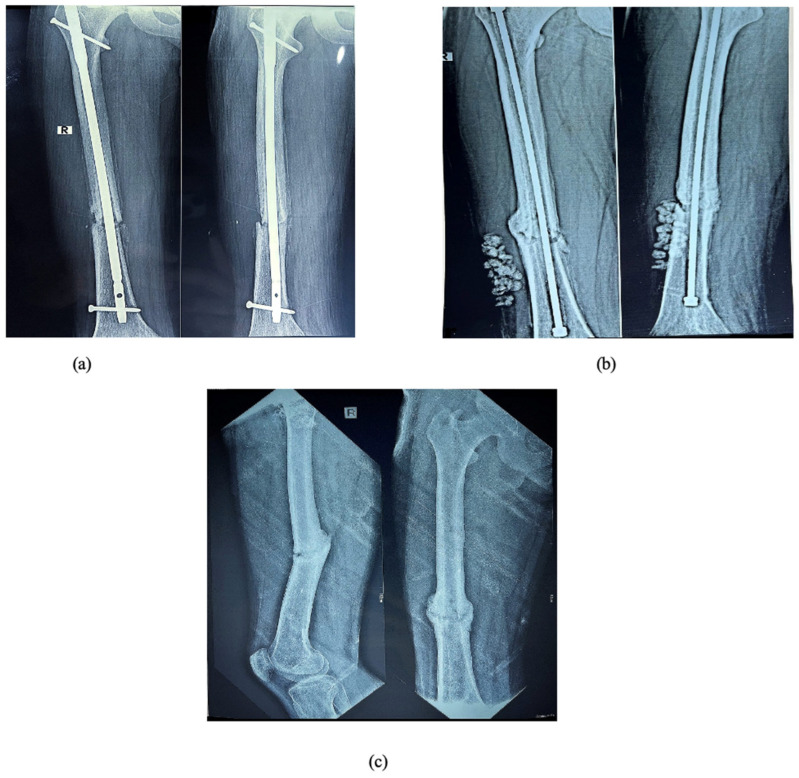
Representative serial radiographs of infected nonunion managed with a custom antibiotic-coated locking nail. (a) Preoperative radiograph showing infected nonunion; (b) third-week postoperative AP and lateral views showing the implant in situ; (c) six-month AP and lateral views showing progressive cortical bridging and radiographic union.
